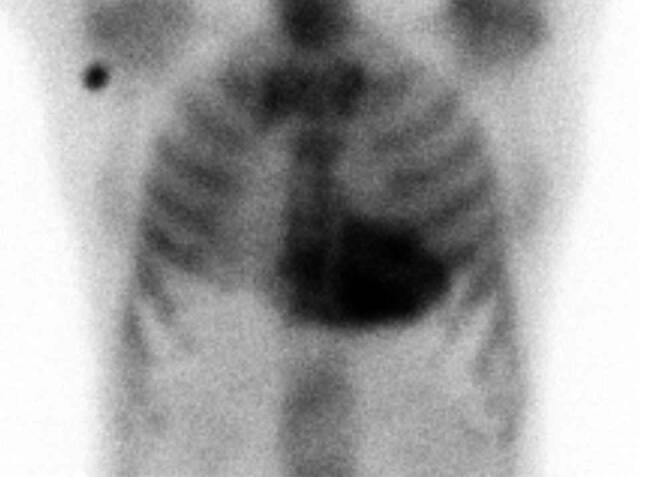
^99m^Tc‑3,3‑diphosphono‑1,2‑propanodicarboxylic acid (99mTc-DPD) bone scintigraphy: anterior planar image demonstrating intense myocardial tracer uptake (Perugini grade 3)
